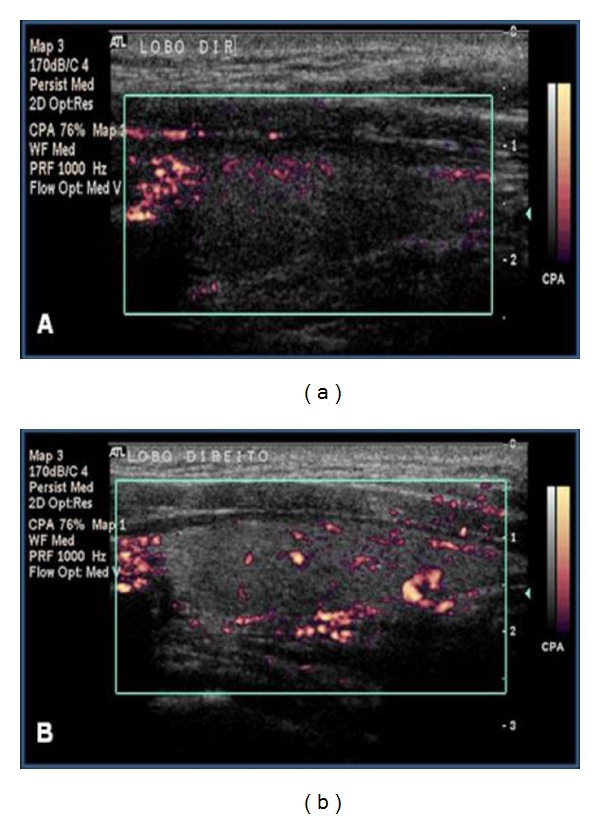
Power Doppler. Thyroid parenchyma vascularization in an L-group patient in whom patterns I pre-LLLT (a) and II post-LLLT (b) were observed.
